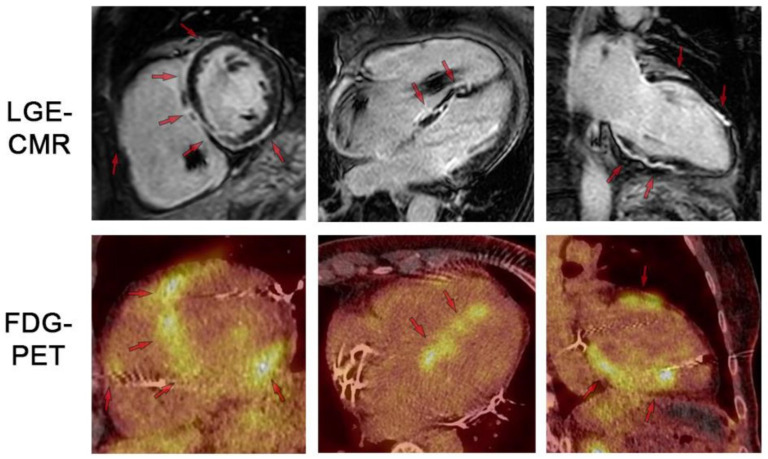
LGE-CMR and FDG-PET images for respective short-axis, 4-chamber, and 2-chamber orientations. Top row shows LGE-CMR fibrosis imaging. Arrows indicate areas of abnormal LGE in a patchy distribution pattern reflecting inflammation and/or scar. Bottom row shows fusion FDG-PET/CT showing patchy uptake in regions of scar, suggesting active inflammation. Note the artifact in the RV cavum caused by the ICD lead. LGE: late gadolinium enhancement. FDG: 18-fluordesoxyglucose positron emission tomography. Reproduced from: Korthals,. D, et al. (2024) [12]. Licensed under CC BY 4.0 (https://creativecommons.org/licenses/by/4.0/ accessed on 15 December 2025).
